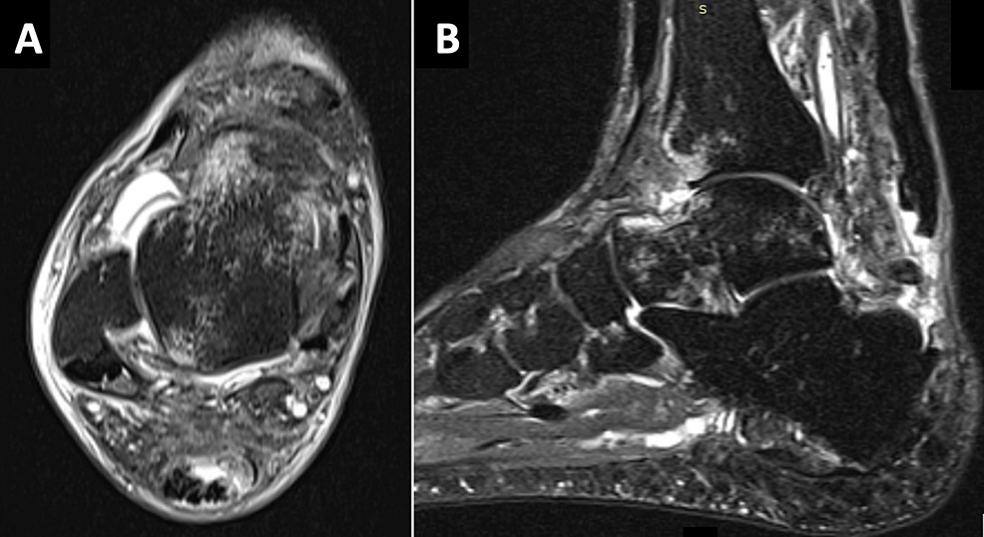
MRI showing an insertion rupture of the Achilles tendon and a rupture of the FHL: (A) T2 MRI axial images, (B) T2 MRI sagittal images FHL: flexor hallucis longus, MRI: magnetic resonance imaging
